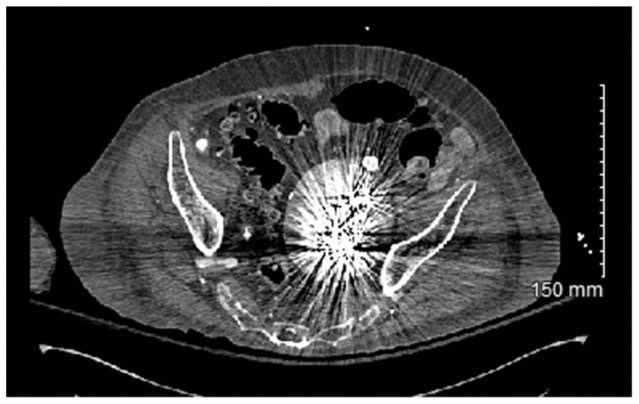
One-month postoperative computed tomography angiography (CTA) demonstrating a stable, thrombosed left internal iliac artery (IIA) aneurysm measuring 8.9 cm, with complete exclusion of type II endoleak.
